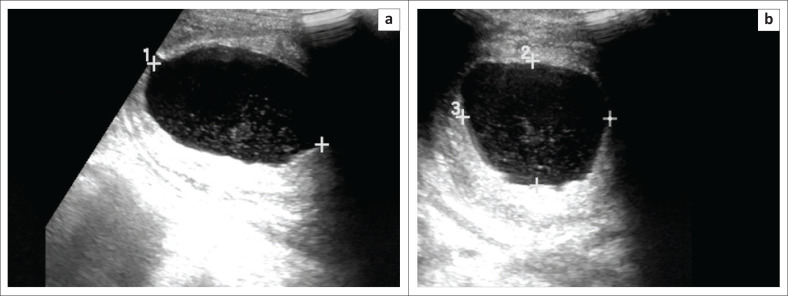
Ultrasound images of the bladder in the sagittal planes (a) and transverse (b) indicate multiple intravesical low-level echoes. These were mobile on real time B-mode scan.
